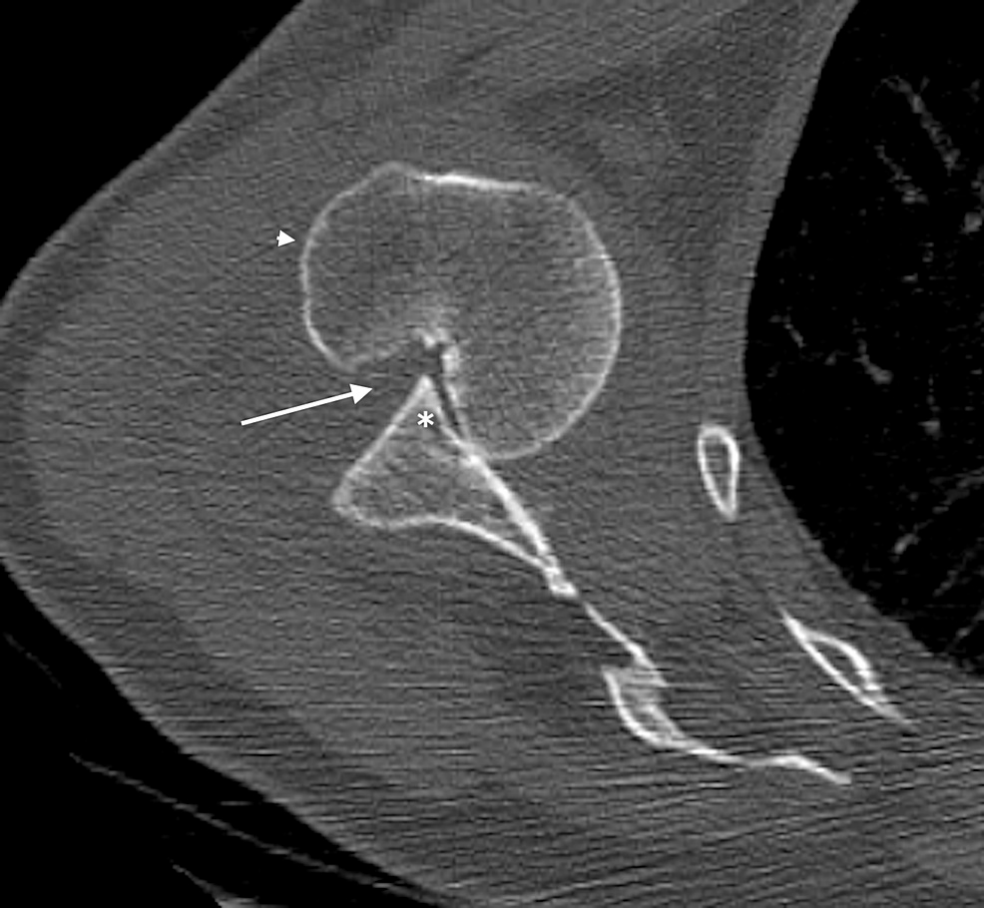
CT scan (axial section) of the left shoulder showing the anteriorly dislocated humeral head (arrow head) locked over the anterior glenoid (asterisk mark) with a large Hill-Sachs lesion (white arrow).
